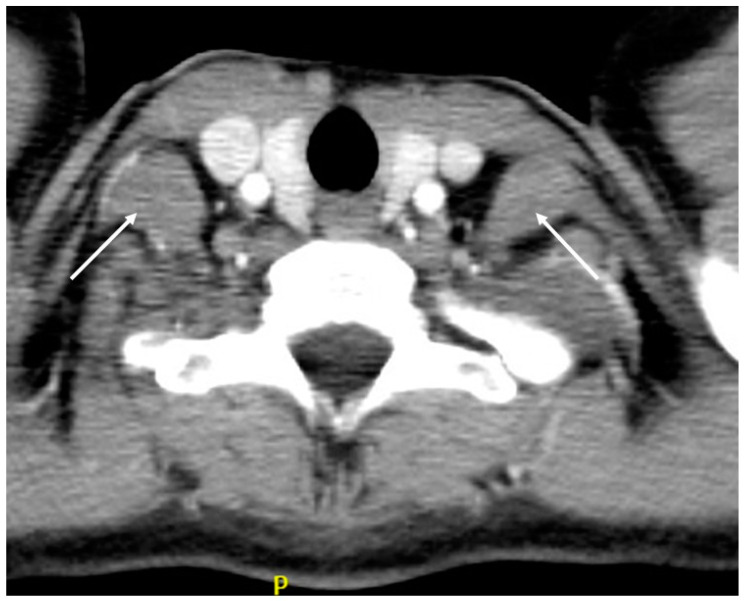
CT scan disclosing bilateral anterior scalene muscle hypertrophy (white arrows).
